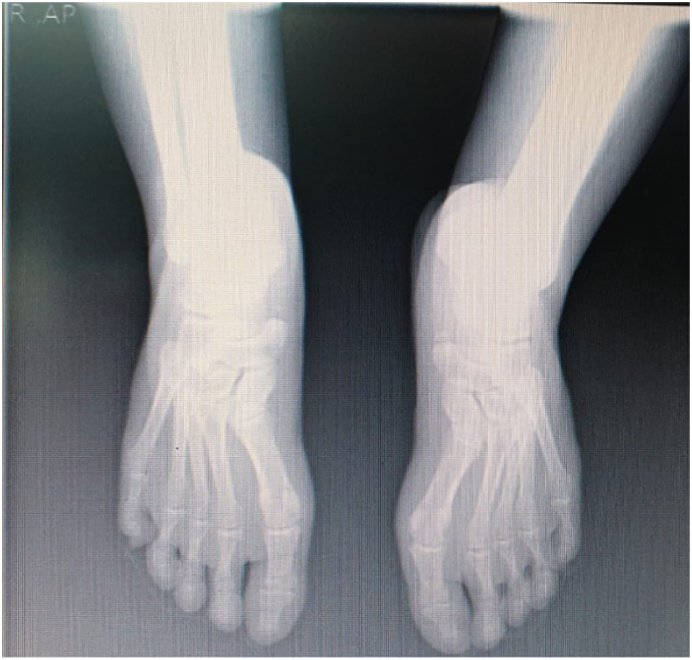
AP/lateral radiography finding.
